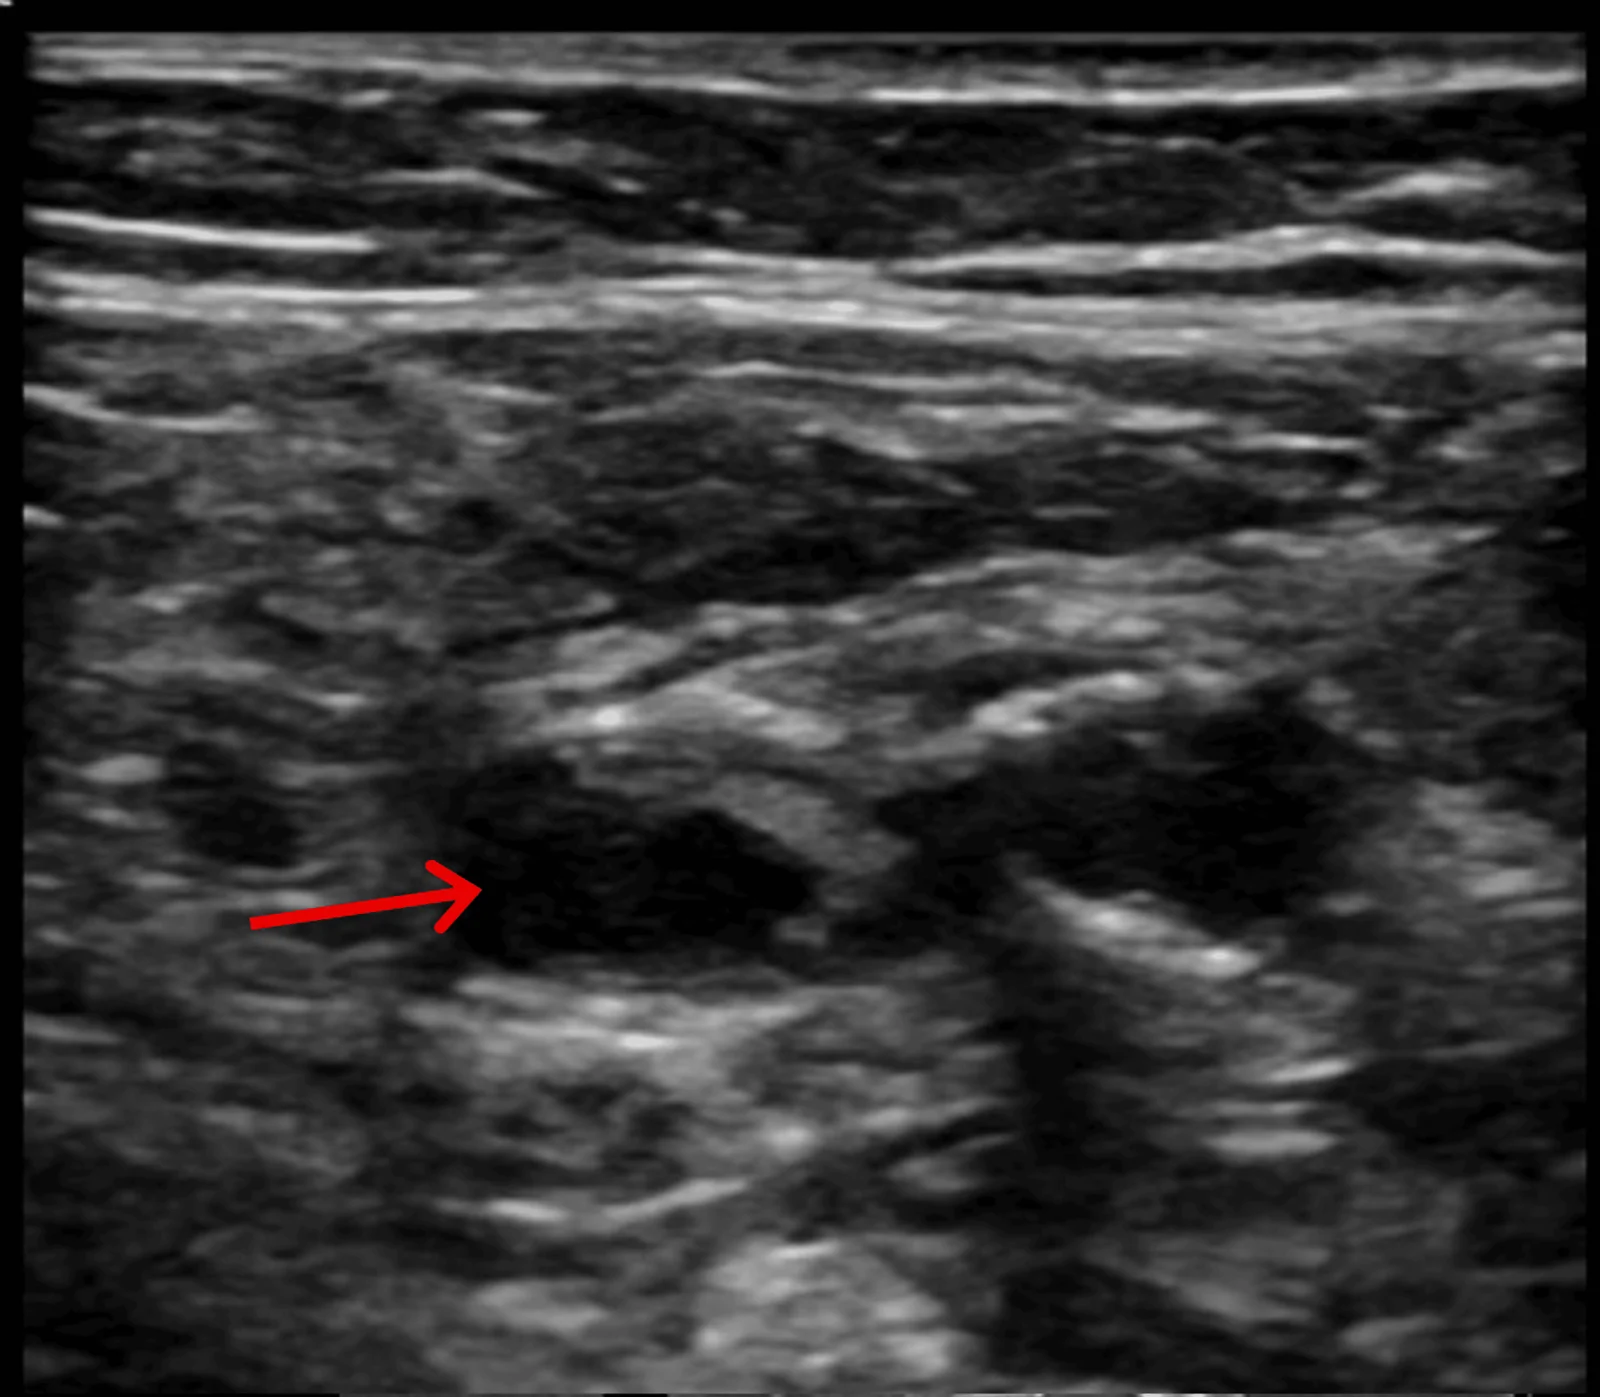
Transversal section of an ultrasonography showing a thrombosis of the left commune femoral vein, indicated by an arrow
